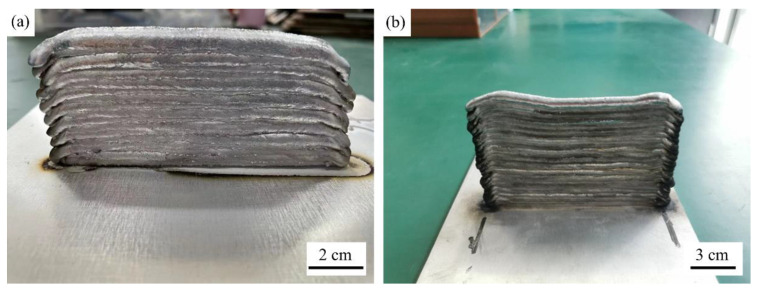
(a) Thin-walled parts fabricated with ultrasonic vibration; (b) thin-walled parts fabricated without ultrasonic vibration.
